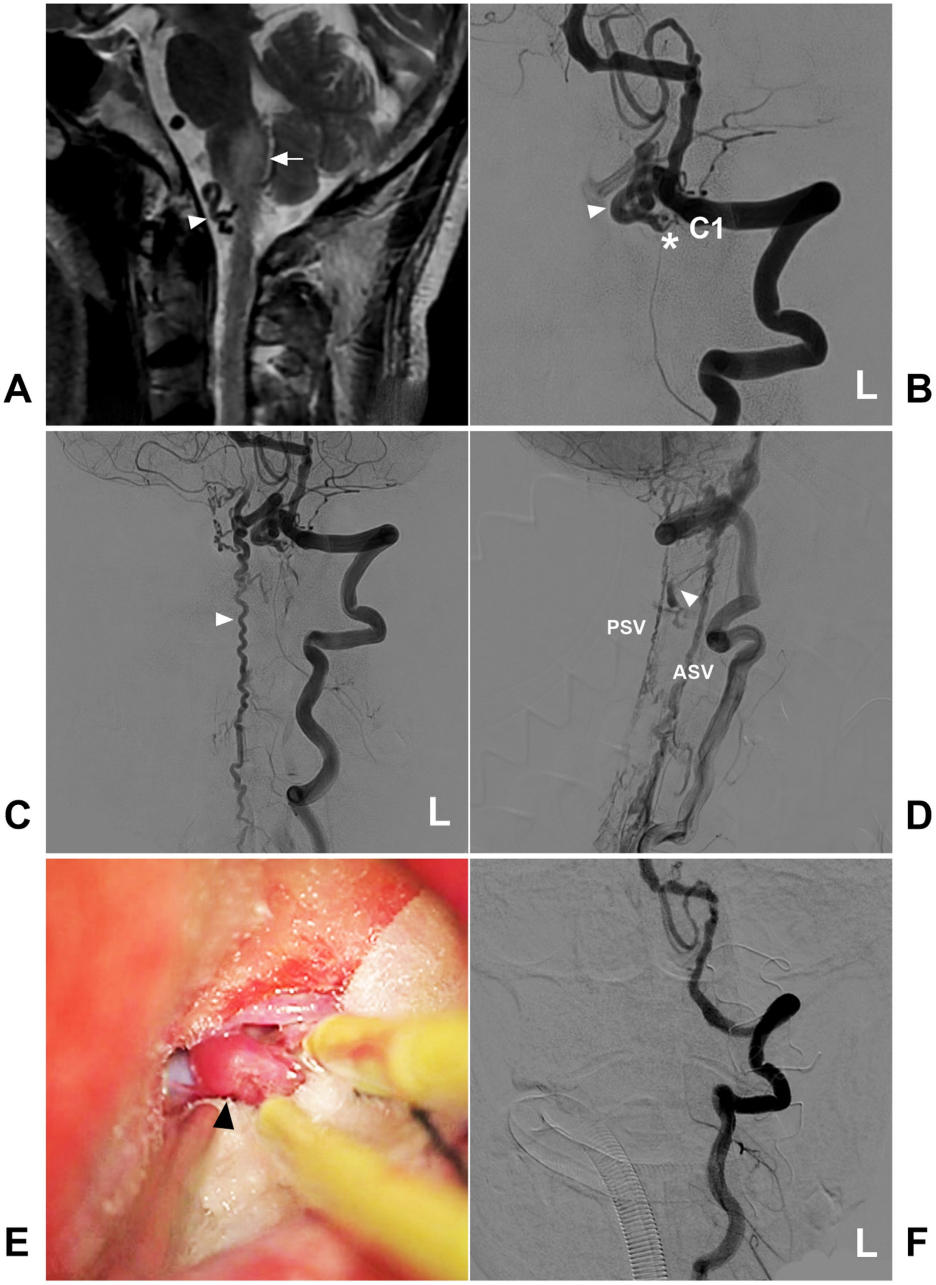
Open surgery in a high cervical CCJ DAVF with downward drainage. (A) MRI showing edema (arrow) of the medulla oblongata and abnormal vessels (arrowhead) in the front of the brainstem. (B) Arterial phase DSA of the left VA showing a CCJ DAVF (asterisk) fed by the C1 radicular artery. The drainage went into the intracranial vein (arrowhead). (C) Late arterial phase DSA of the anterior posterior view showing downward drainage into the perimedullary vein (arrowhead). (D) Late arterial phase DSA of the lateral view showing downward drainage, including downward ASV and PSV, and lateral drainage by the bridging and radicular veins (arrowhead). (E) Intraoperative image showing the thick arterialized draining vein (arrowhead). (F) Postoperative DSA showing that the DAVF was obliterated after the draining vein was coagulated and cut. ASV, anterior spinal vein; C1, first cervical vertebra; CCJ, craniocervical junction; DAVF, dural arteriovenous fistula; DSA, digital subtraction angiography; L, left; MRI, magnetic resonance imaging; PSV, posterior spinal vein; VA, vertebral artery.
